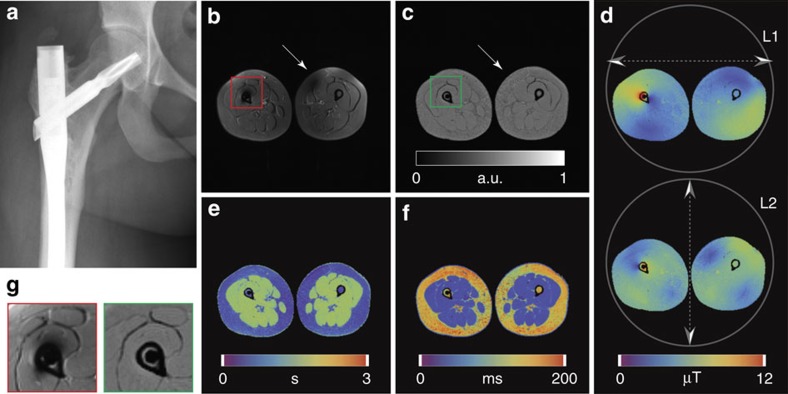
Conventional MRI versus PnP-MRF in the presence of an orthopaedic implant. (a) X-ray image showing the orthopaedic implant. (b) Contrast-weighted axial image through the legs obtained using a conventional inversion recovery (IR) TSE sequence. Signal voids appear both in the vicinity of the implant and in the contralateral leg. (c–f) Quantitative maps obtained using PnP-MRF, including PD, B_1_^+^, T1 and T2, respectively. Units are arbitrary for PD (c), micro Tesla for B_1_^+^ (d), seconds for T1 (e) and milliseconds for T2 (f). (g) Enlargements, extracted from b (red frame) and c (green frame), comparing the region surrounding the implant with conventional (left) and PnP-MRF (right) approaches. (PnP-MRF scan time: ∼28 s per slice.) Note the absence of B_1_^+^-related signal voids in any of the PnP-MRF parameter maps.
